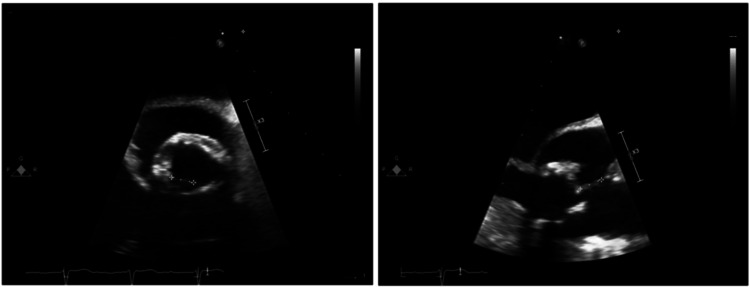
Transesophageal echocardiogram showing a mobile, echogenic vegetation attached to the non-coronary cusp of a prosthetic aortic valve Image credit: Puxeddu et al. [18] (CC BY 4.0)
